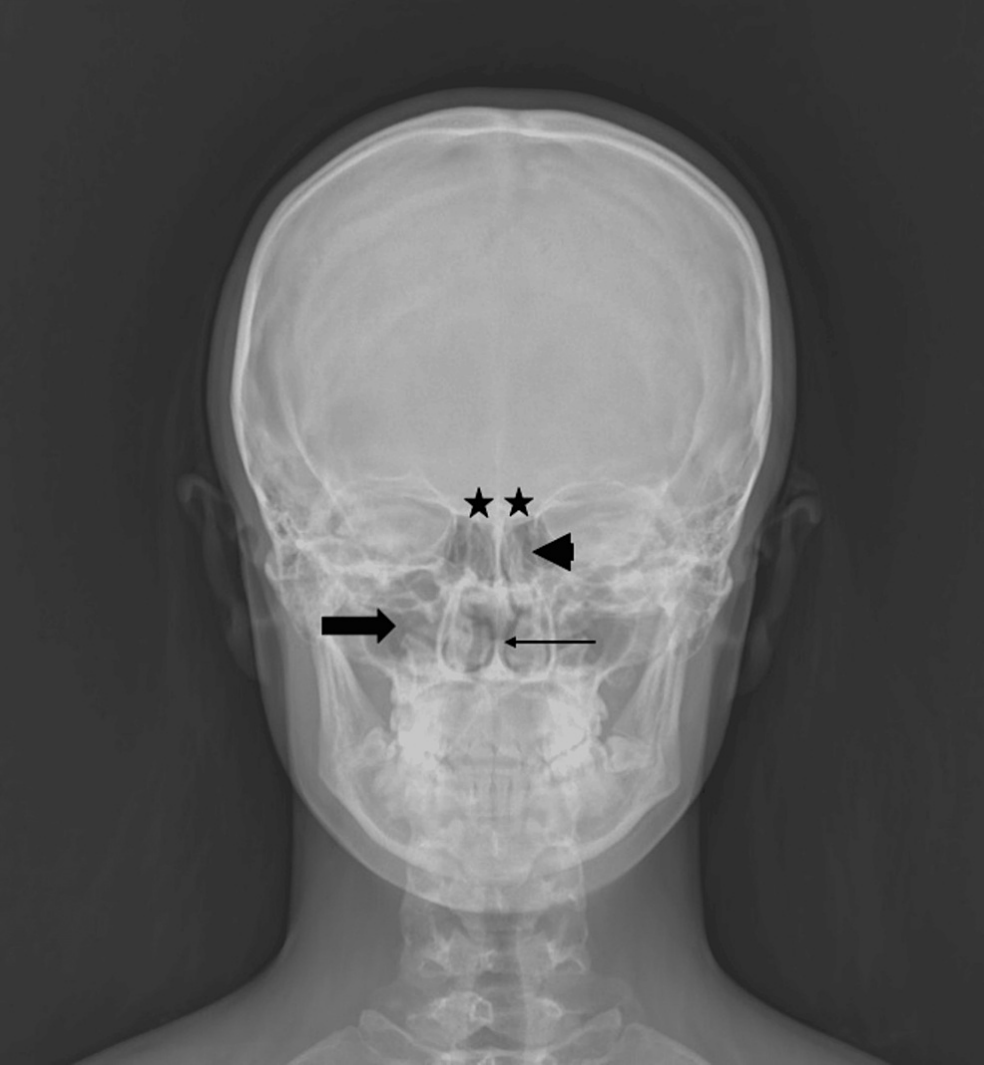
Radiography image of a 20-year-old woman with bilateral frontal aplasia. The image shows bilateral frontal aplasia (star), maxillary sinus (bold arrow), ethmoidal cells (arrowhead), and nasal septum (arrow).
